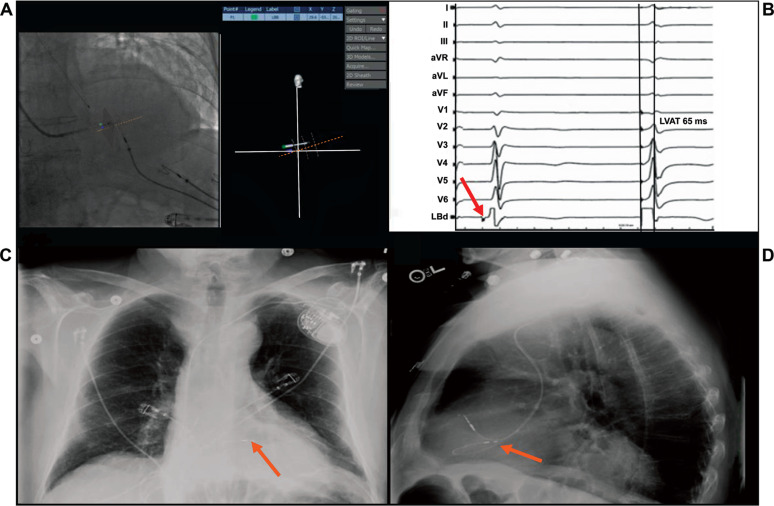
Use of a three-dimensional mapping device to guide left bundle branch area pacemaker implant. A: Fluoroscopy image on Navik 3D showing the left bundle branch pacing area on the septum and right lead trajectory (orange dashes). B: Electrogram showing the left bundle potential at lead location (red arrow) and paced beat with left ventricular activation time of 65 ms. C and D: Two-view chest X-ray postimplant showing lead location (orange arrow).
